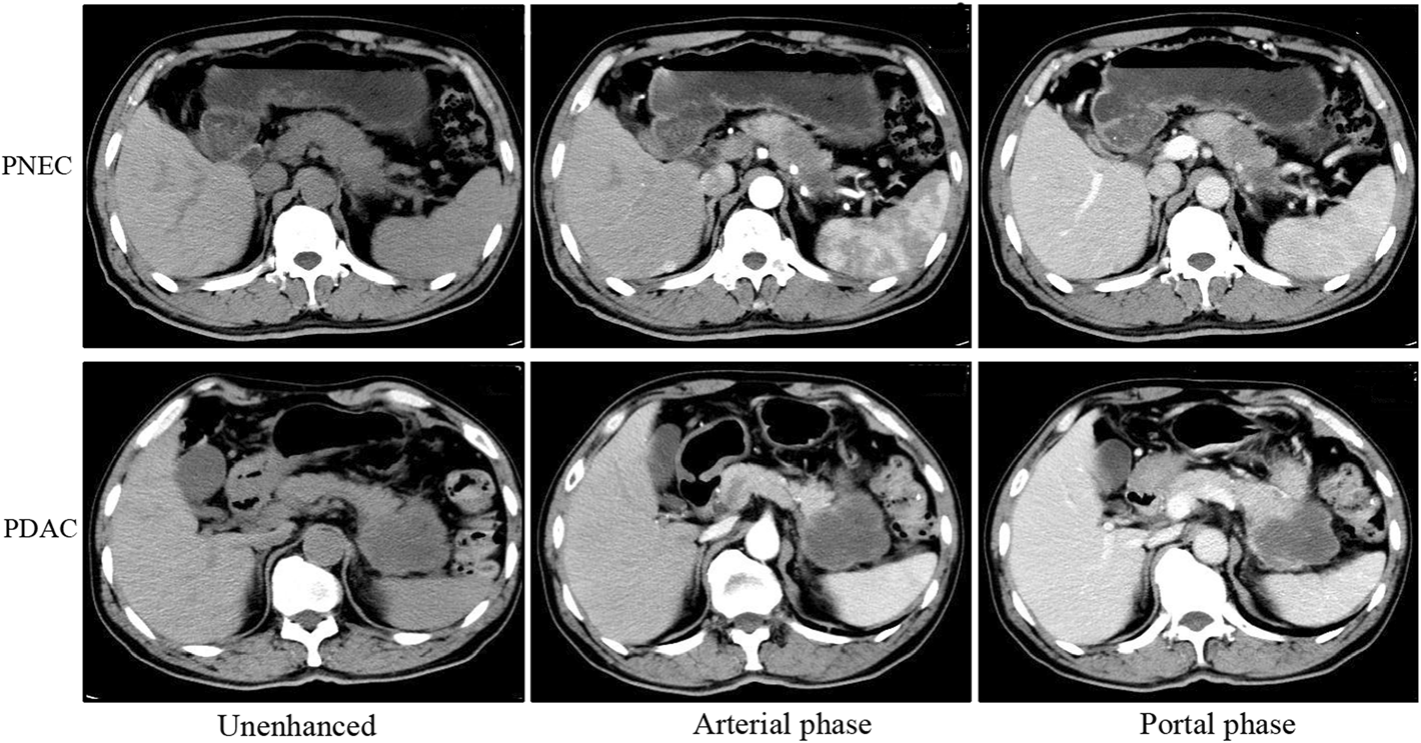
The computed tomography imaging findings in a 66-year-old woman with pancreatic neuroendocrine carcinoma (PNEC, white arrow) and a 62-year-old man with pancreatic ductal adenocarcinoma (PDAC, black arrow). Unenhanced and contrast-enhanced CT images at the arterial phase and portal phase showed ill-defined, hypovascular mass
